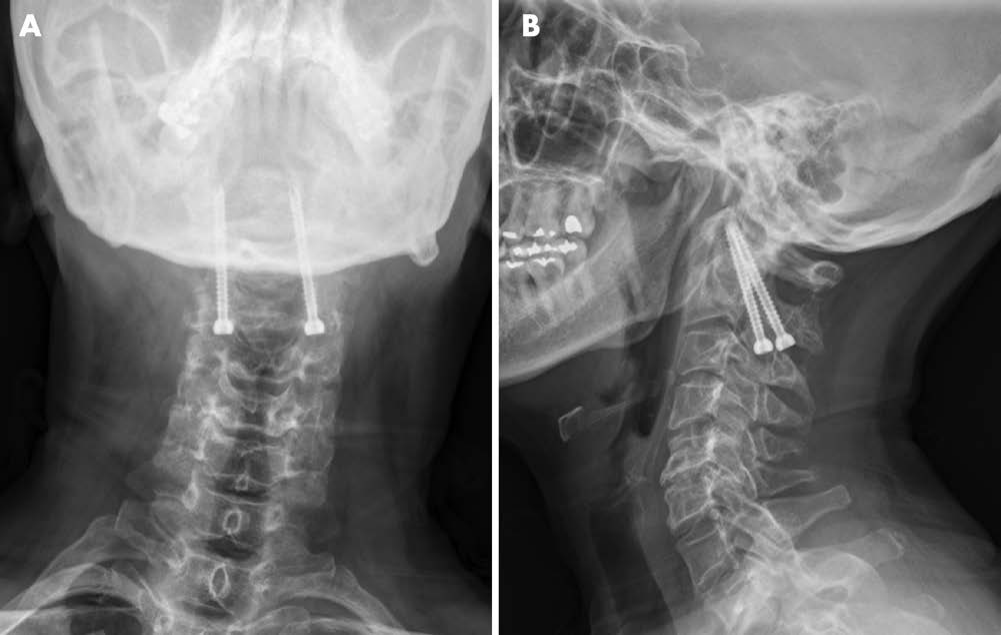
(Case 2) A postoperative plain lateral radiograph shows an intact alignment and implant.
